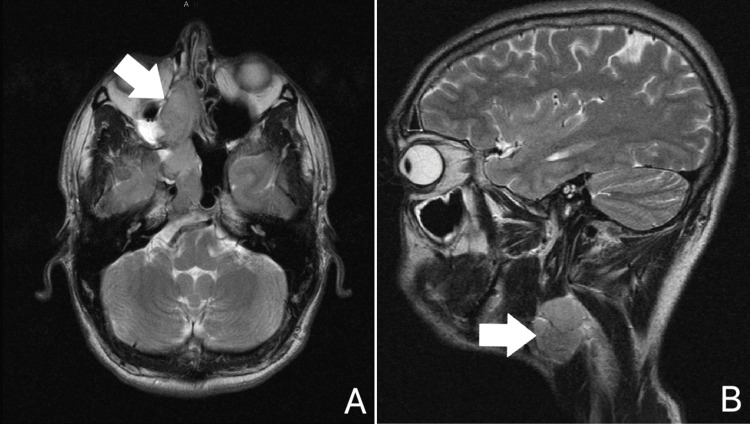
Axial MRI scan of the brain with intravenous contrast. Axial MRI scan of the brain with intravenous contrast demonstrating a lesion measuring 55x26x46 mm occupying the right nasal cavity (white arrow - panel A). Sagittal view displaying a right submandibular lymph node block (white arrow – panel B).
